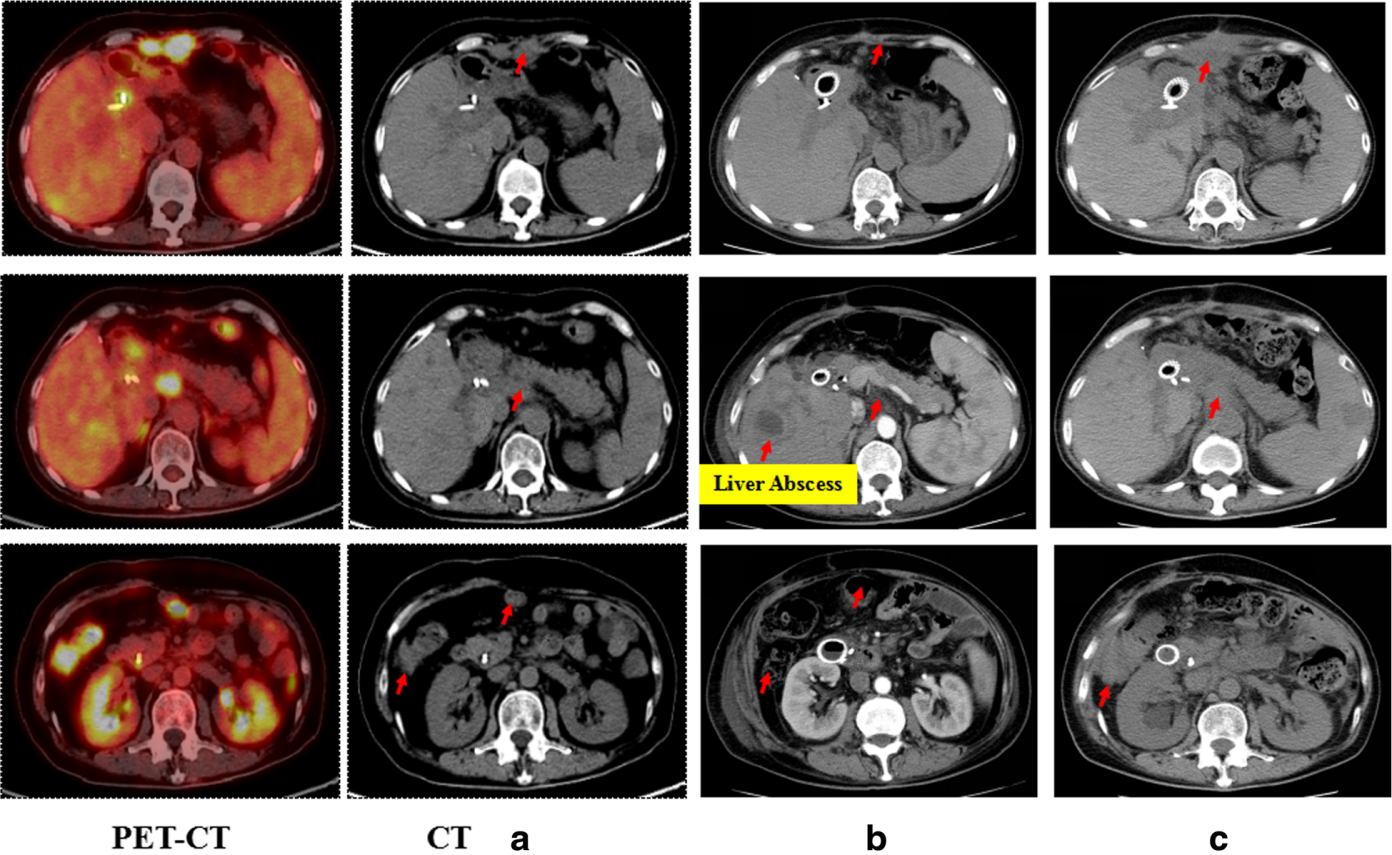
Outcome of CART133 cell infusion. (*a*) With the guidance of PET, tumor lesions were labeled on the images presented by computed tomography (CT) scans before the infusion of CART133 cells (*red arrow*). (*b*) CT images showed remarkable shrinkage or even disappearance of some metastases in the peritoneum and abdominal cavity (*red arrow*) and an abscess in the right liver. (*c*) CT scan detected an approximate 40 × 70 mm new metastatic lesion under the abdominal wall and suspected metastases in the abdominal cavity 4.5 months after the CART 133 immunotherapy (*red arrow*)
